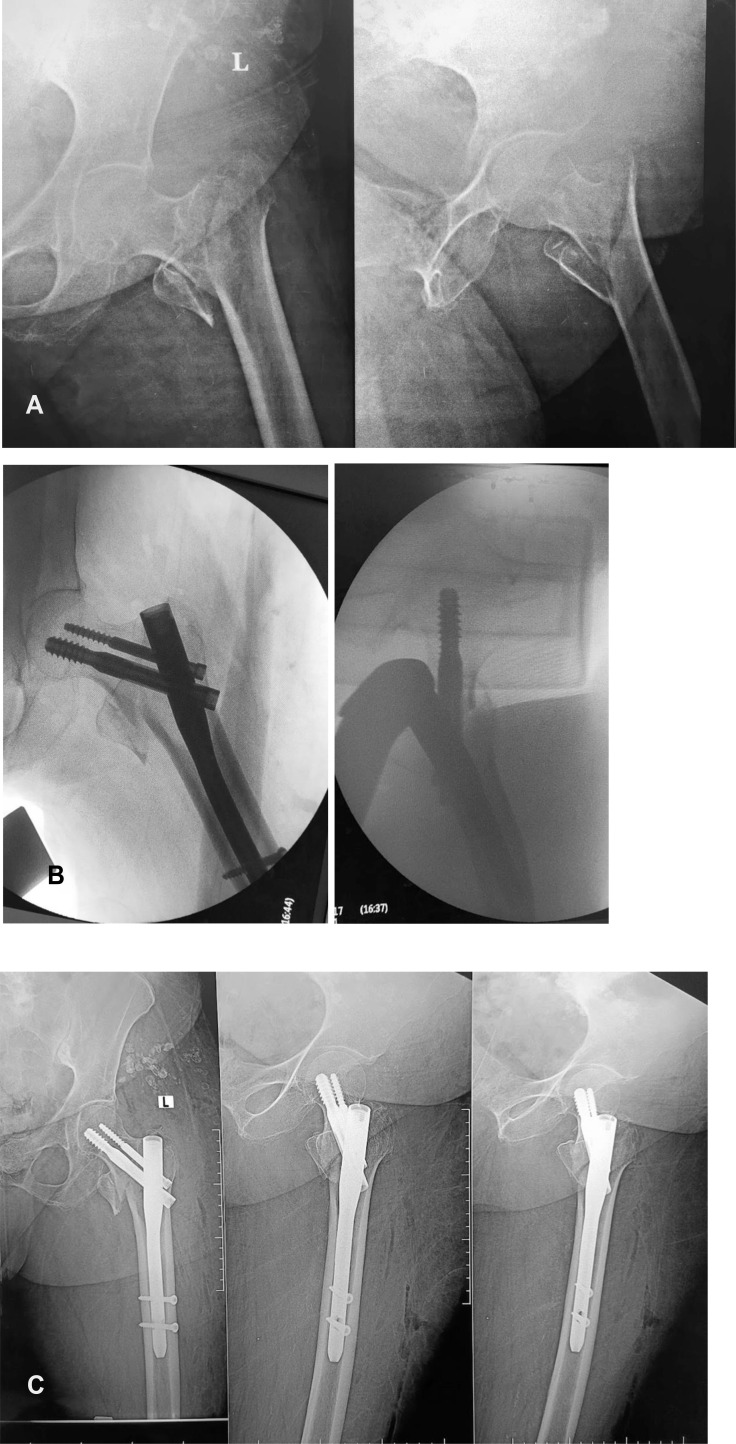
The case of a patient with an intertrochanteric femoral fracture managed by proximal femoral nailing using a traction table in the supine position. (A) Preoperative X-ray of a 71-year-old woman who presented with an unstable trochanteric fracture. (B) Intraoperative fluoroscopic photos showing the position of the proximal locking screws (lag screw and antirational screw). (C) Postoperative X-ray image of the proximal femur showing the position of the nail and the central position of the lag screw, the TAD is less than 25 mm, CDA is about 135°, and position of proximal locking screws according to the Cleveland and Bosworth quadrants in quadrant number 5, and the quality of reduction was good according to the CRQC.
